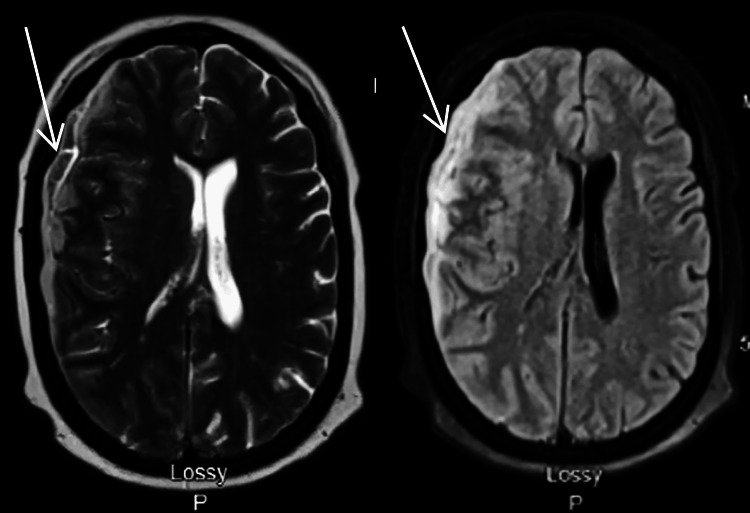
Contrast-enhanced brain MRI prior to surgery, demonstrating a thick-walled, peripherally enhancing, enlarging subdural collection overlying the right frontotemporal regions.
